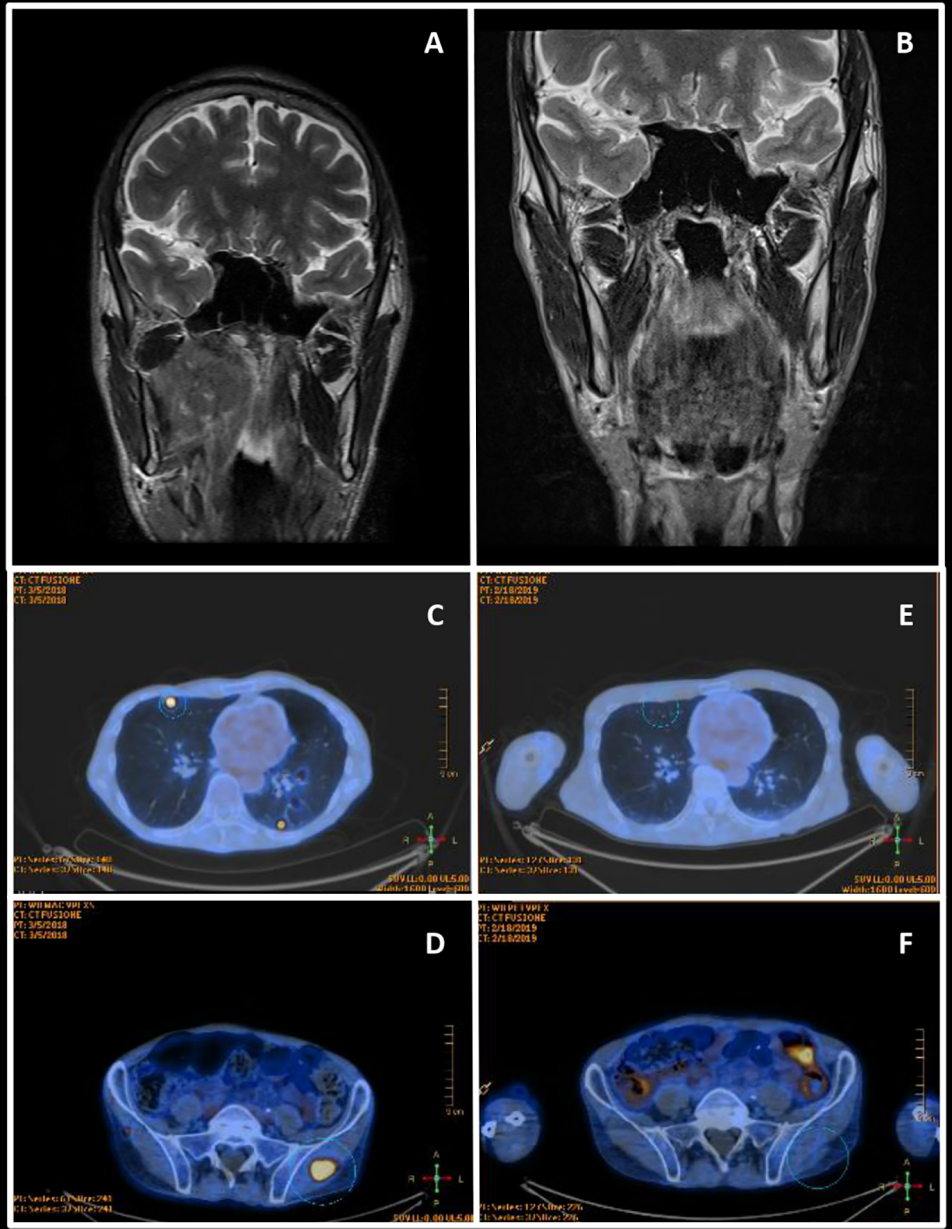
Radiological assessments. (A) March 2017 Head and neck contrast-enhanced magnetic resonance imaging—before the primary treatment. (B) November 2017 Head and neck contrast-enhanced magnetic resonance imaging of complete remission after chemoradiation. (C, D) March 2018 Whole body FDG PET—at diagnosis of metastatic disease. (E, F) November 2018 Whole body FDG PET of complete response after nivolumab.
